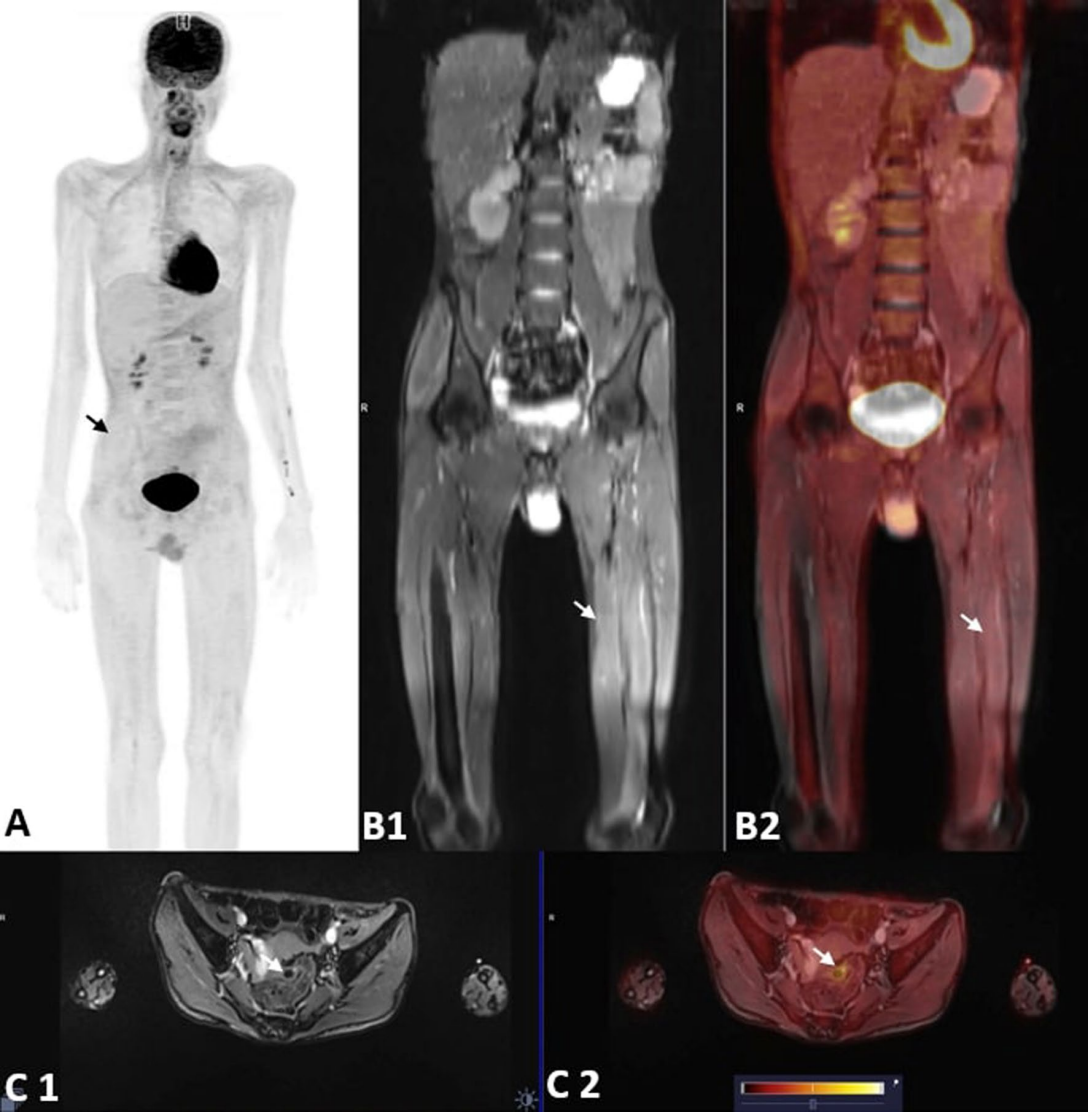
PET-MRI images of a 16-year-old boy with Overlap Myositis. A Whole body MIP image (black arrow). B1 Coronal T2 FS BLADE MR image showing hyperintensity changes in the muscles around hip and lower limbs (white arrow). B2 Coronal fused PET/MRI image shows increased FDG uptake in all the muscles at HIP and lower limbs (white arrow). C1 and C2. Axial T2FS BLADE MR showing subtle hyperintensity changes in the gluteal muscles (white arrow). Axial Fused PET/MR images showing mild diffuse increased FDG uptake in gluteal muscles (white arrow)
